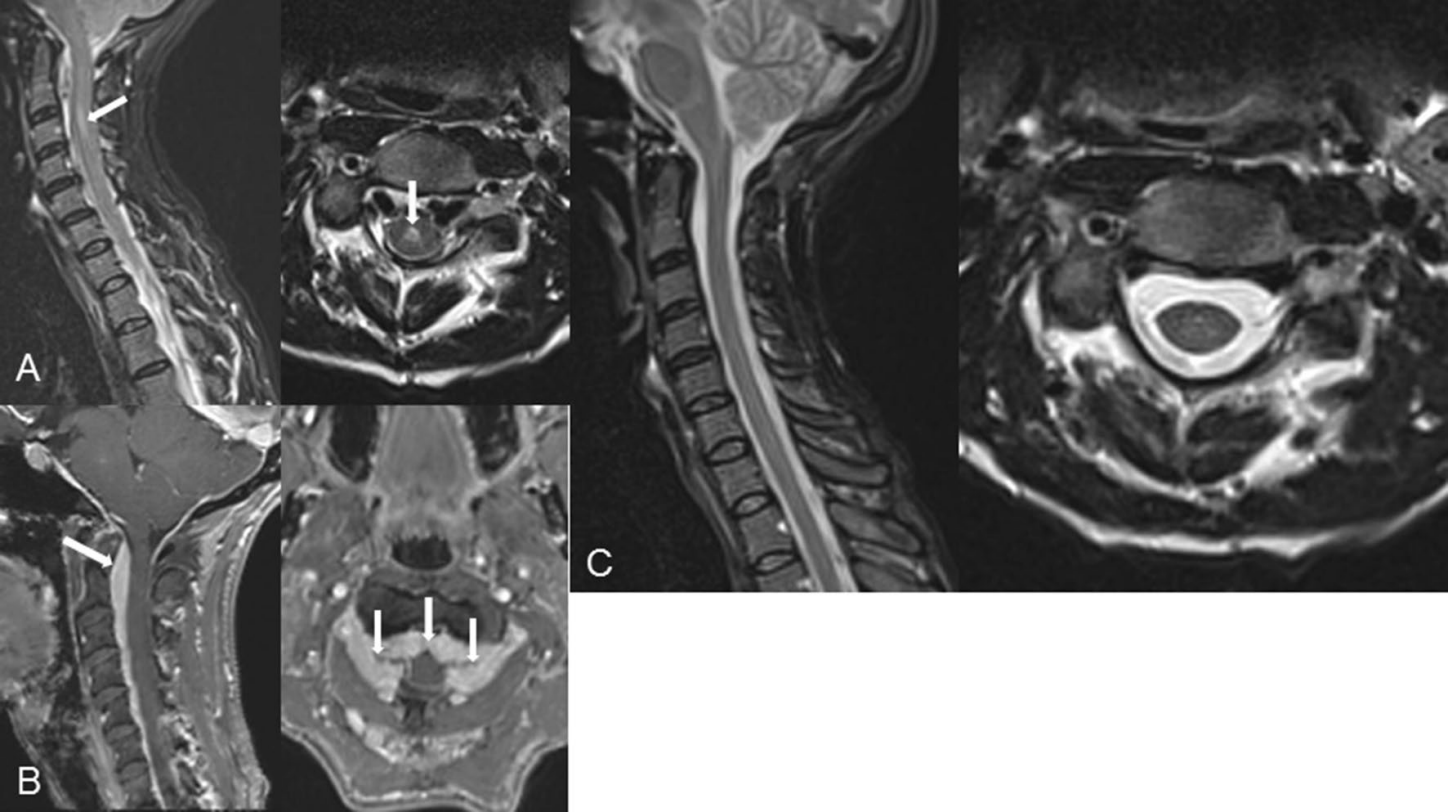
a Preoperative myelomalacia (arrows) at C3 in sagittal STIR-sequences and axial T2 weighted MRI; b Preoperative CE-MRI showing the prominent epidural veins leading to a spinal cord compression (arrows); c Normal epidural space without evidence of persisting myelomalacia at C3 according to the sagittal postoperative STIR- and axial T2 weighted MRI
